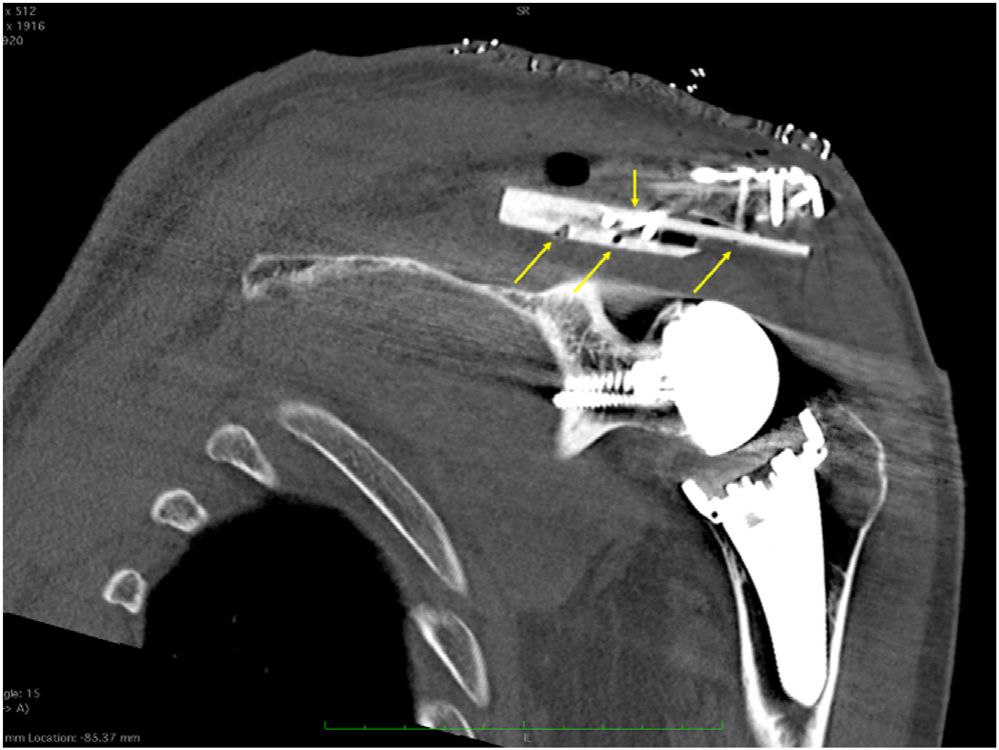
Coronal view of a left shoulder on computed tomography. The fibular allograft, fixed with screws (yellow arrows), offers support under the acromion.
